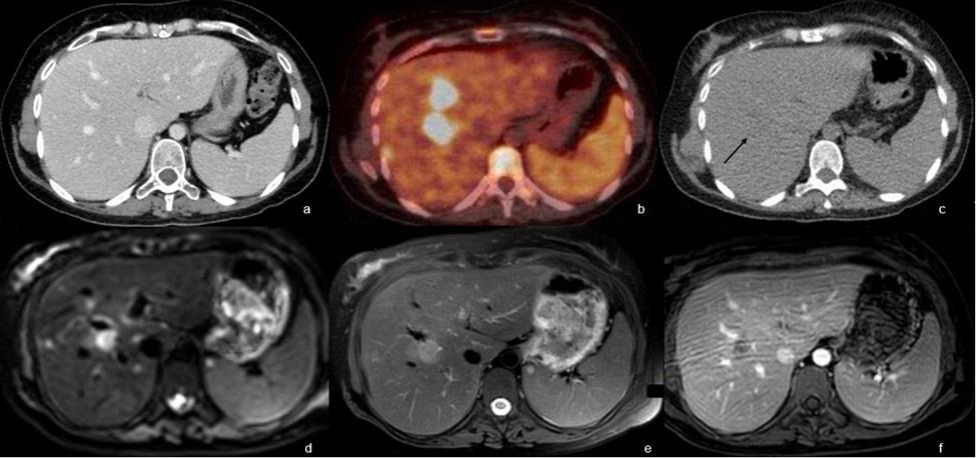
Axial enhanced CT scan of the abdomen shows no abnormality in the abdomen (a). Axial PET/CT fusion image of the abdomen shows few FDG-avid liver lesions with SUVmax 24 (b). Non-enhanced CT scan shows subtle hypodense lesions in the liver (black arrow) (c). Ill-defined lesion in segment VIII showing restriction of diffusion on DWI (d), on T2WI (e) and showing subtle rim enhancement and peri-lesion enhancement on T1WI post gadolinium administration (f)
